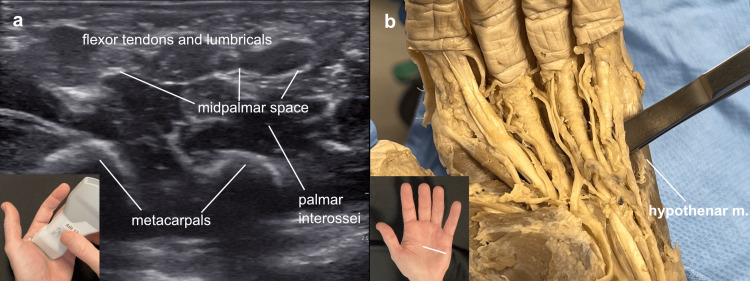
Midpalmar space Midpalmar space: (a) Ultrasound view of a left hand with the probe marker oriented to the left for ease of cadaver correlation, demonstrating the midpalmar space superficial to the interossei, (b) midpalmar space in the cadaver was entered with blunt dissection between the hypothenar muscles and the flexor tendons.
